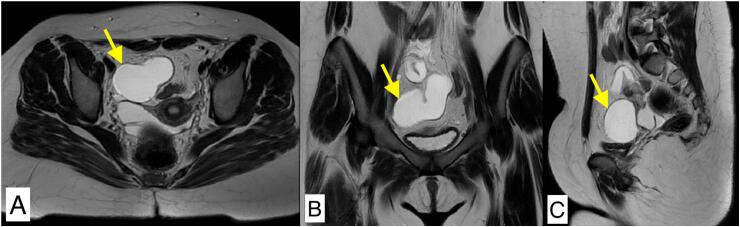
Magnetic resonance imaging (MRI) of pelvis. T2 weighted (A) Axial, (B) Coronal and (C) Sagittal images showing tubular, dilated, convoluted fluid filled structure in right adnexa separate from right ovary.
